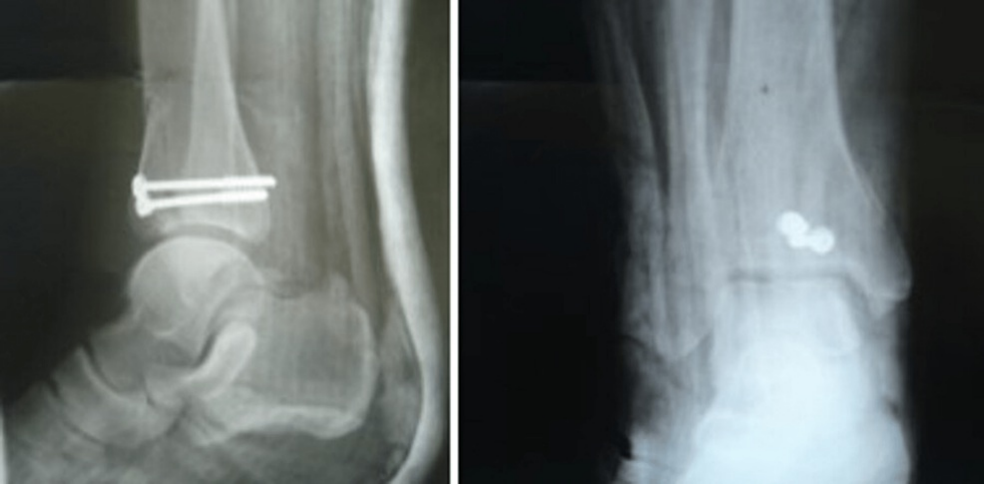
Anteroposterior and lateral postoperative (14 days) X-ray
